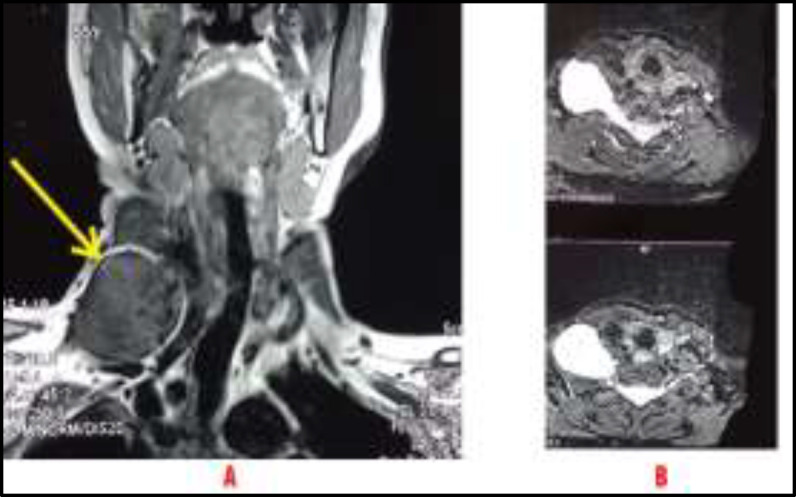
MRI Neck And Chest: Well defined solid cystic lesion in right supraclavicular region: (A) Isointense on T1WI; (B) Heterogenous enhancement seen on post contrast scan
